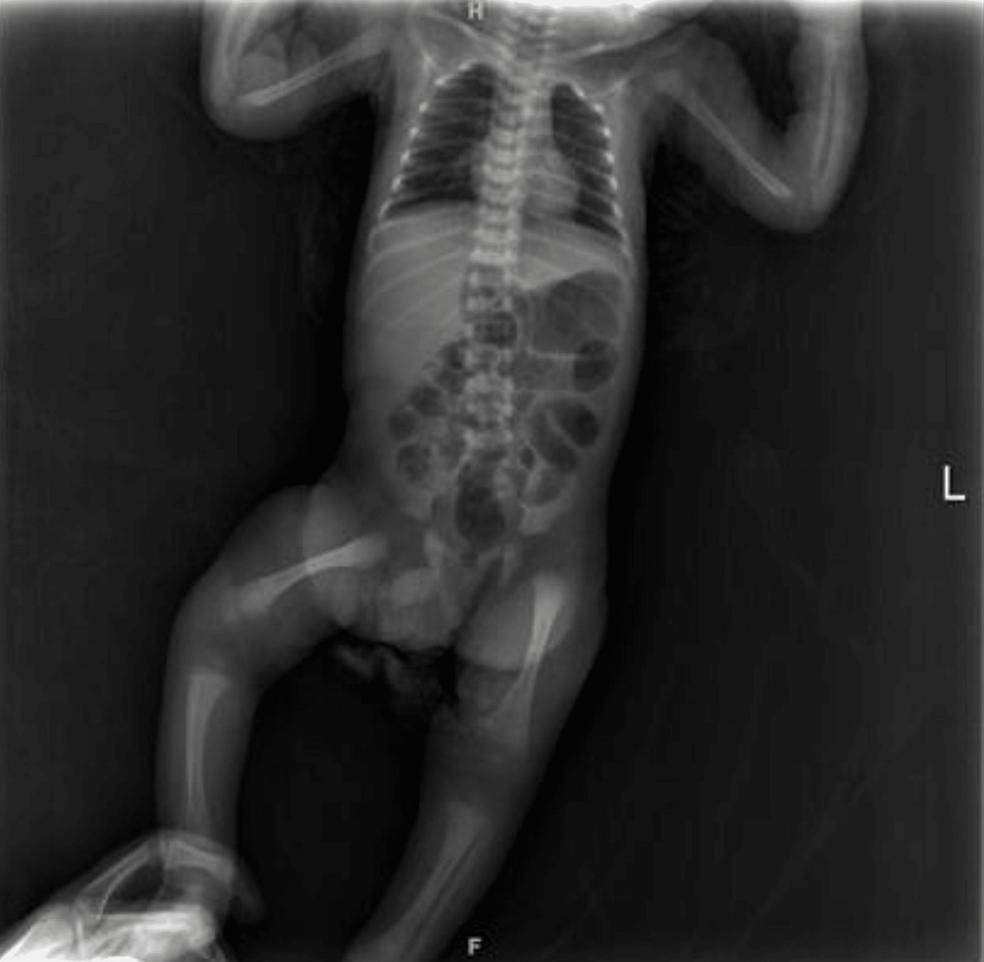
The hip x-ray (anteroposterior (AP) view) showed no findings.
